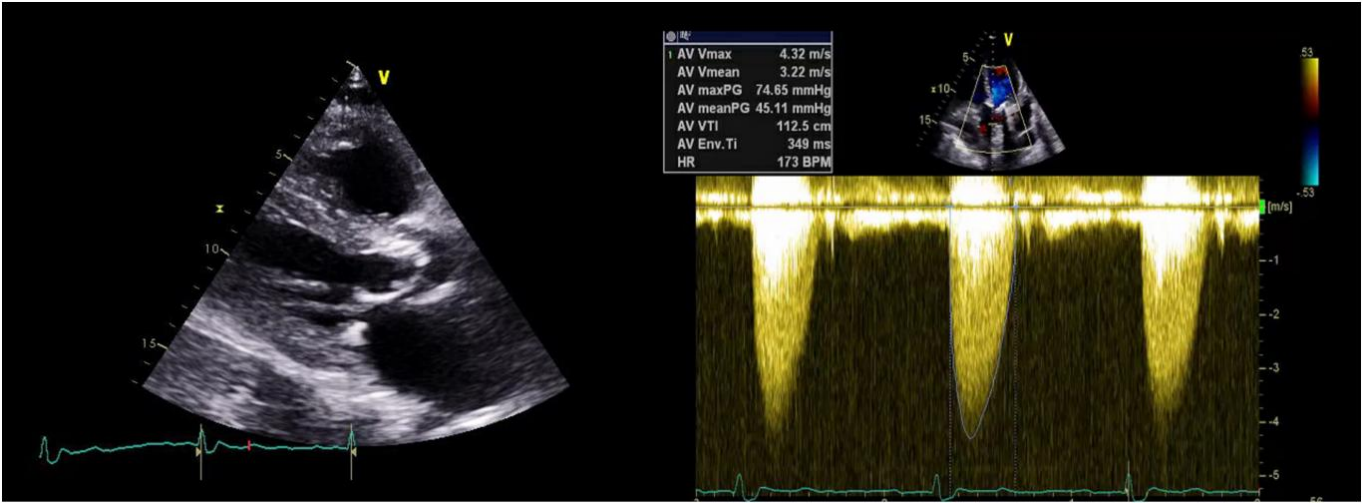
A long-axis echocardiographic image and continuous wave Doppler demonstrating severe aortic stenosis.
